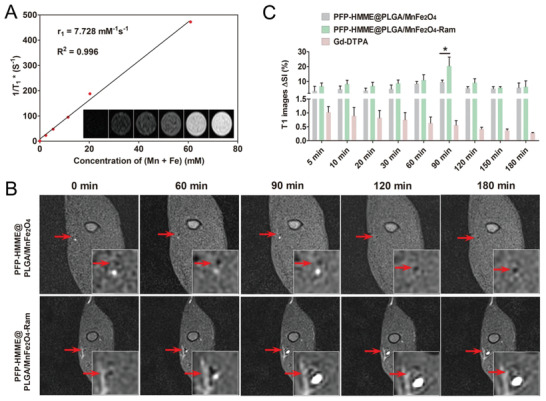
In vitro and in vivo MRI. A) In vitro MRI T1 contrast images and *R*
